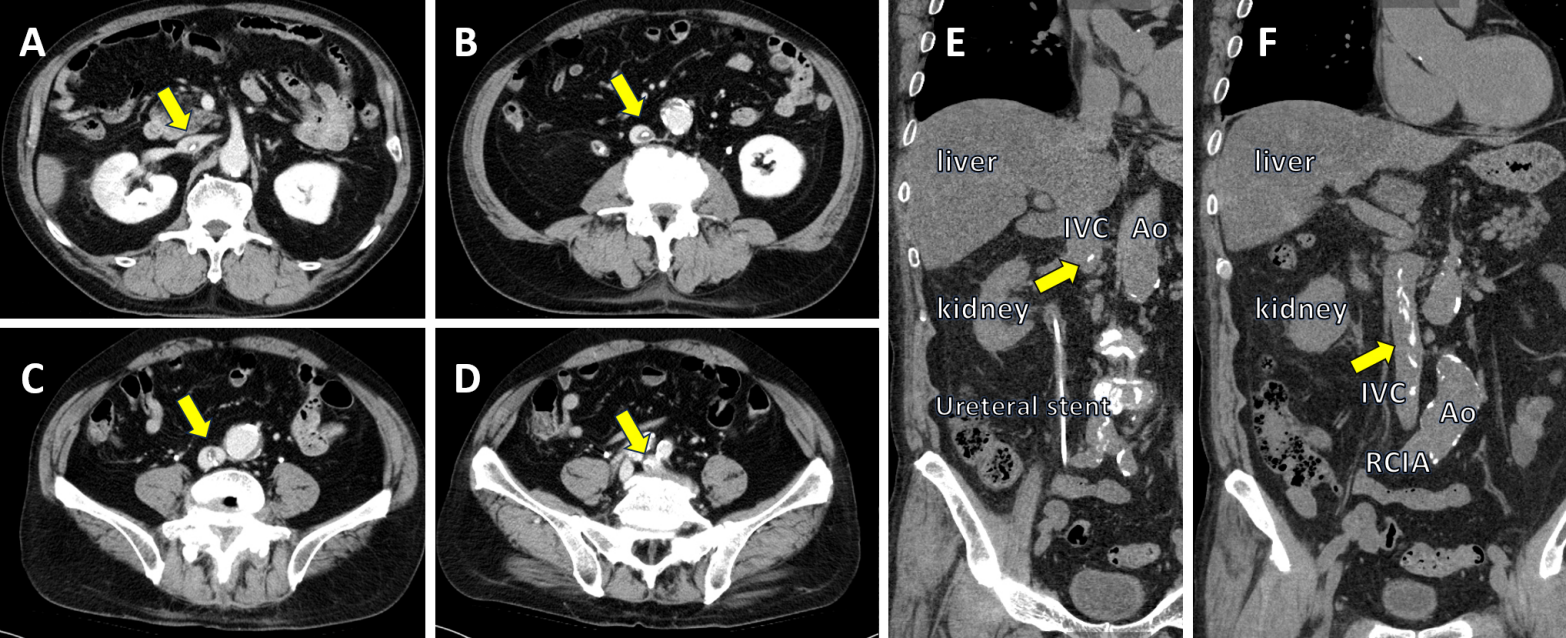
Contrast-enhanced computed tomography showing a calcified thrombus (yellow arrow) extending from the inferior vena cava to the left common iliac vein in axial images (A - D) and coronal images (E, F).
